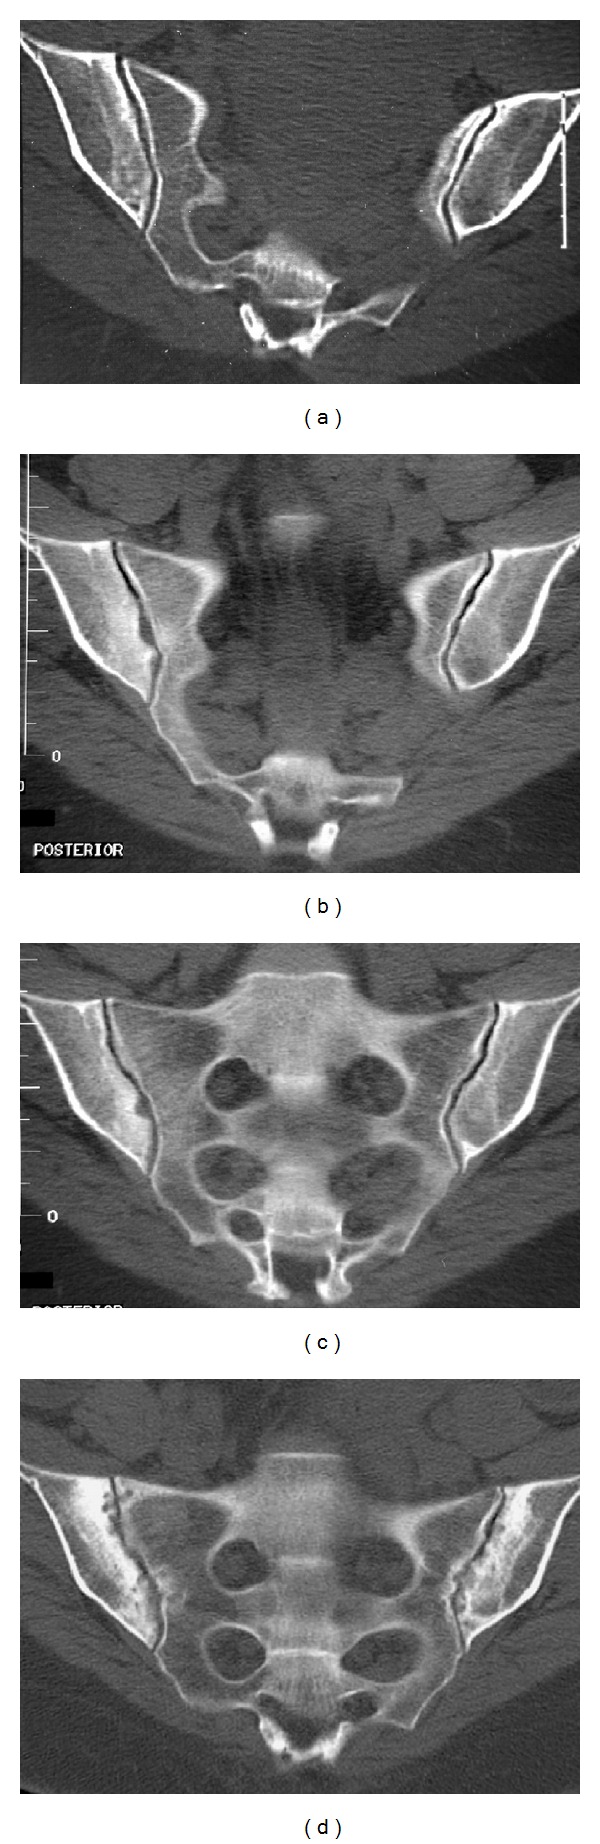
A female patient with three studies showing progression from equivocal to unilateral to advanced bilateral sacroiliitis. (a) The initial equivocal CT, obtained at age 26, shows mild asymmetric sclerosis on the iliac side of the right sacroiliac joint, with a suggestion of a single erosion and slightly poor definition of the iliac subchondral cortex. (b, c) After two years, the suspected changes have progressed into definite unilateral sacroiliitis, with broad erosions and dense inflammatory sclerosis surrounding the right sacroiliac joint. (d) After an additional 11 years, the disease has progressed into bilateral advanced sacroiliitis, with widespread erosions and inflammatory sclerosis affecting both the sacral and iliac sides of the joints.
